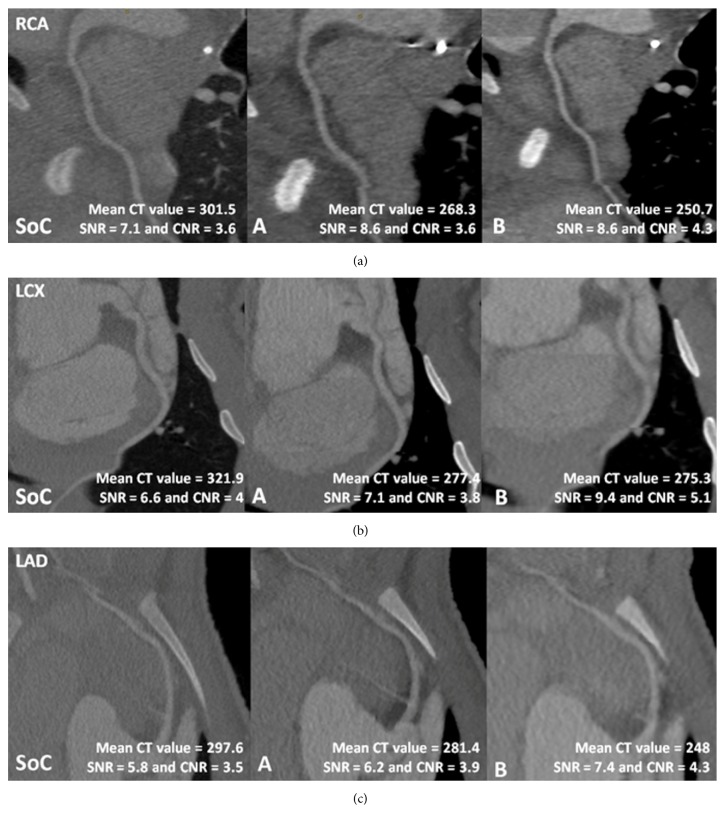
Images of the standard of care protocol and study protocols A and B with the mean CT value, SNR, and CNR of the right coronary artery (RCA) (a), left circumflex artery (LCx) (b), and the left anterior descending artery (LAD) (c).
